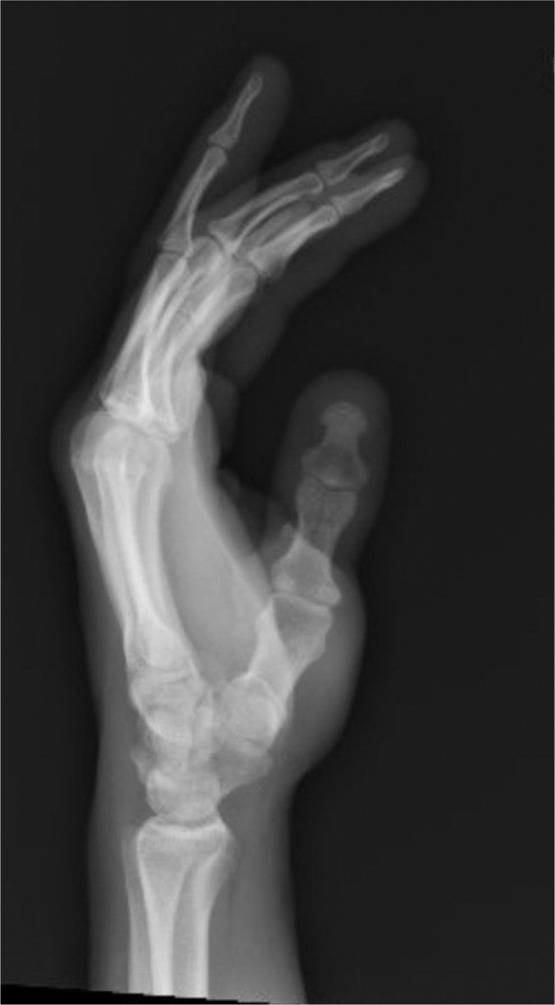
Lateral X-Ray of right hand (Case 2: Pre-op).
